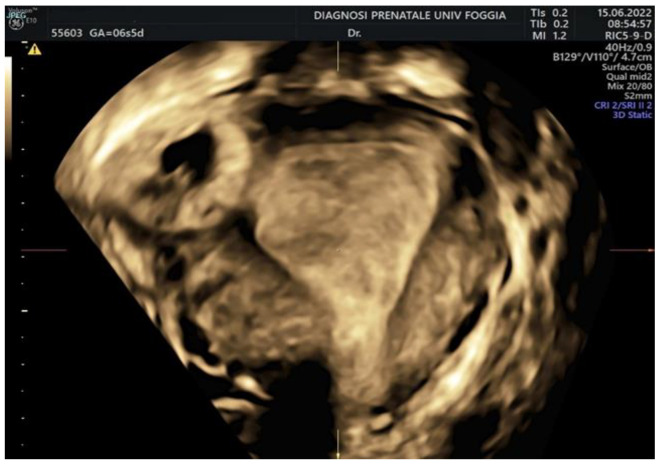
3D transvaginal ultrasound showing the interstitial pregnancy.
